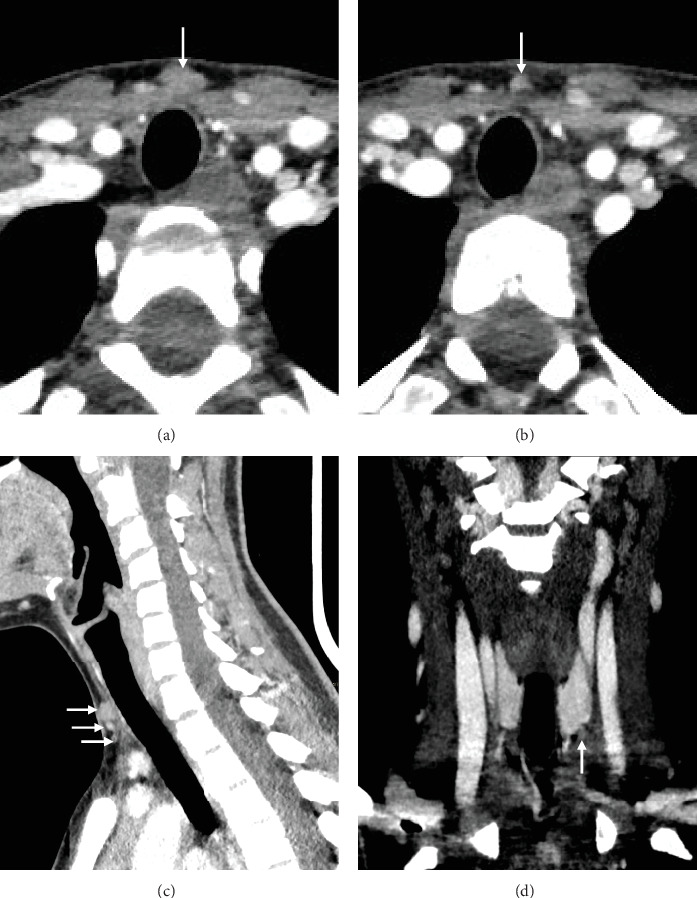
Recurrent hyperparathyroidism 2 years after parathyroidectomy. 4D computed tomography in the transverse (A, B), sagittal (C), and coronal (D) planes showing mildly enhancing nodules in the pretracheal subcutaneous tissue (arrows in subparts (A–C)) and at the lower pole of the left thyroid lobe (arrow in subpart (D)). Size ranges between 3 and 12 mm.
